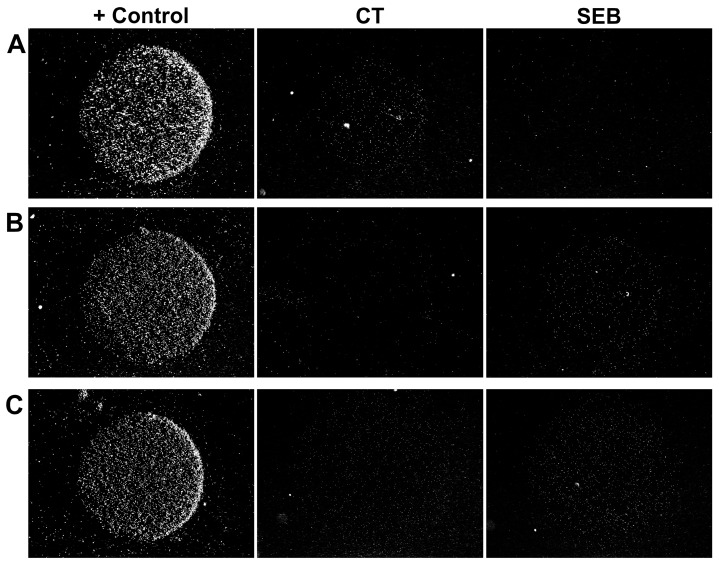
Representative examples of microarray images. The microarray comprised two specific spots with the specificity shown at the top and one positive control spot. (A,B) Individual toxins CT (A) and SEB (B), 0.1 fM each; signal accumulation time: 5 min. (C) Simultaneous detection of CT and SEB at 0.1 fM each; signal accumulation time: 8 min. Each image shows a 1.3 × 1 mm^2^ area.
